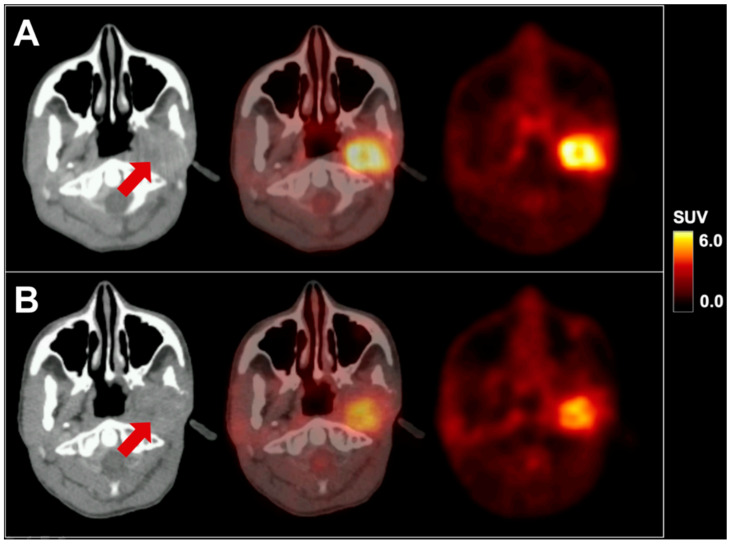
^18^F-FDG PET/CT in axial planes of a pediatric sebaceous carcinoma before (A) and after therapy (B). The red arrow indicates the primary site in the left parotid gland. SUV = standardized uptake value.
